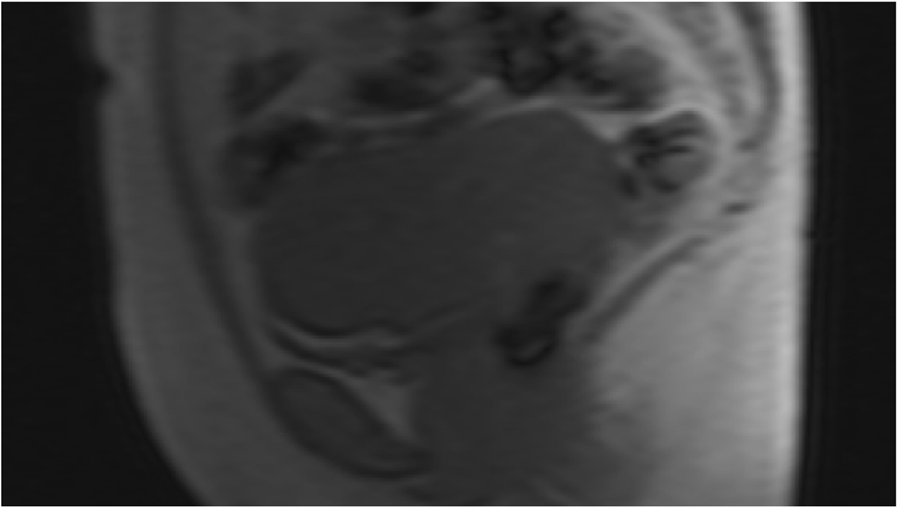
Magnetic resonance imaging studies
